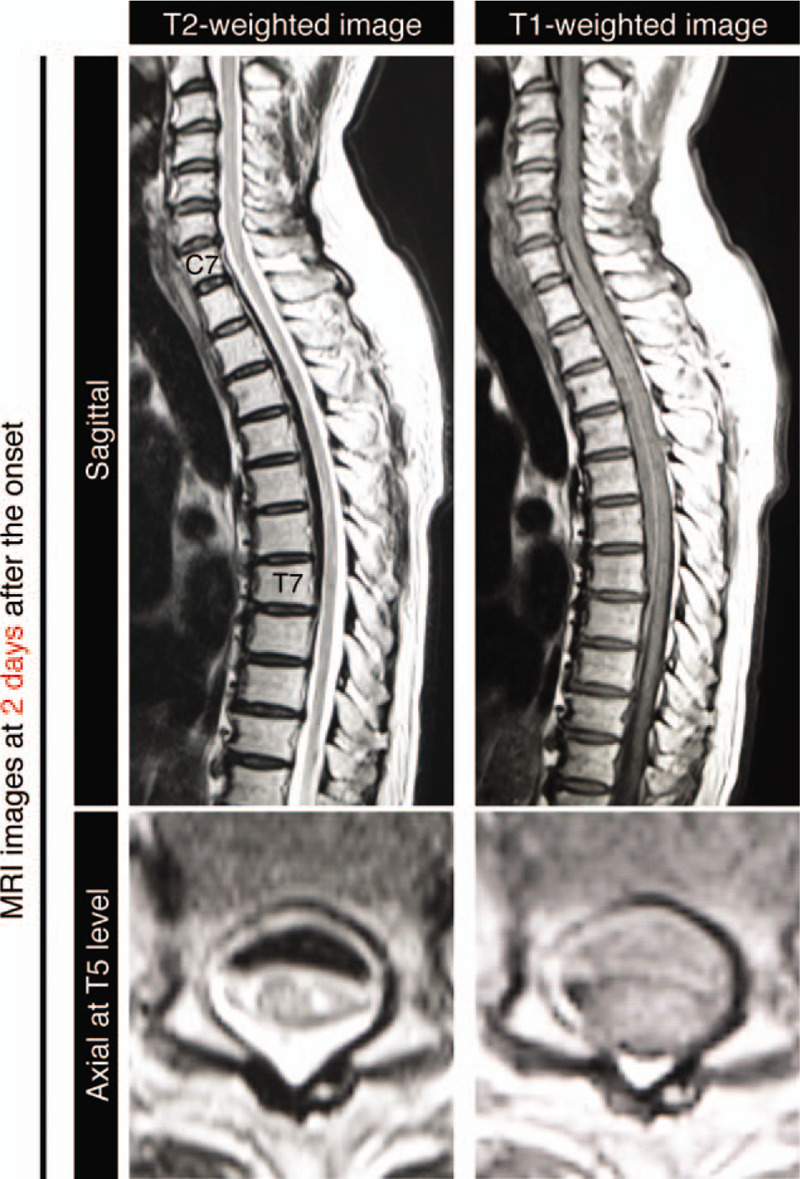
MRIs of the cervicothoracic spine at two days after the onset of presenting paraplegia. T2-weighted and T1-weighted images revealed that the compression of the spinal cord was released from the hematoma. The intensity of the lesion showed hypointensity in the T2-weighted images. MRI images of an axial section at the T5 level also showed the mitigated displacement of the spinal cord due to the hematoma.
